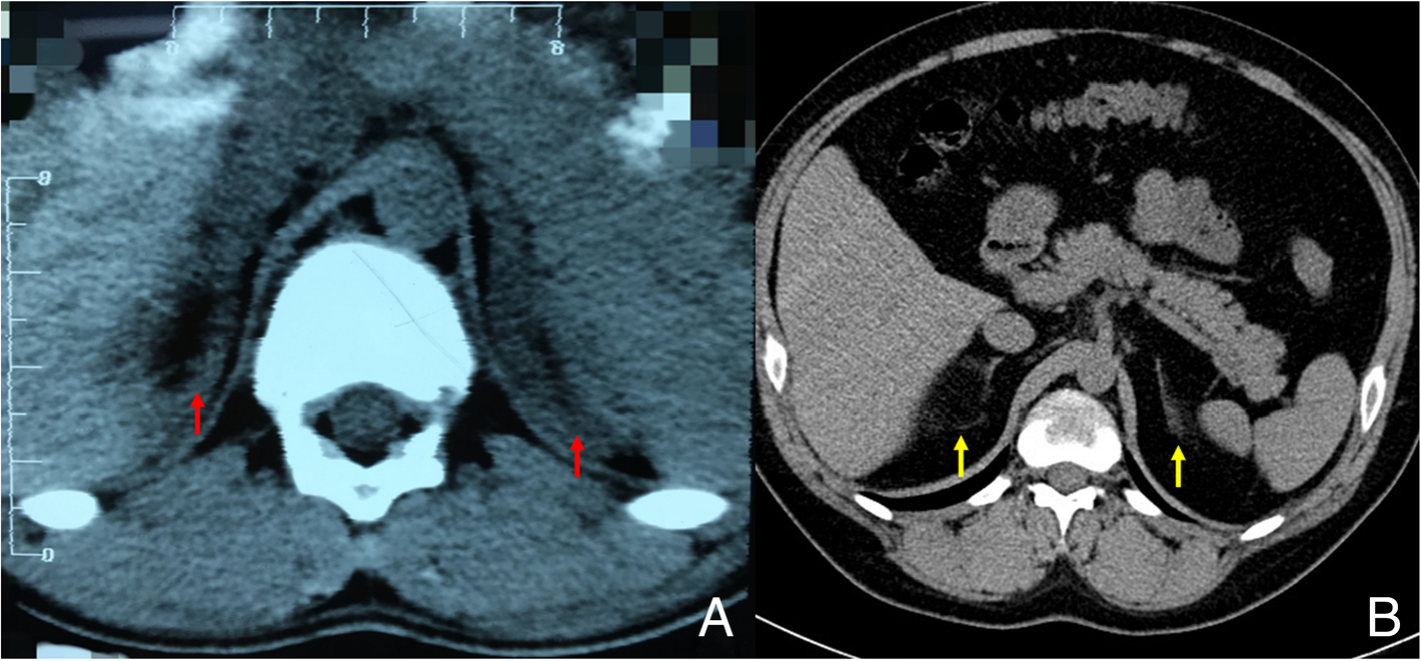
Adrenal computed tomographic scan. a 13 years ago (age: 7 years, 3 months), before treatment, the scan revealed bilateral adrenal hyperplasia (red arrow). b On admission (age: 19 years), after treatment with dexamethasone, the scan showed bilateral adrenal atrophy (yellow arrow)
